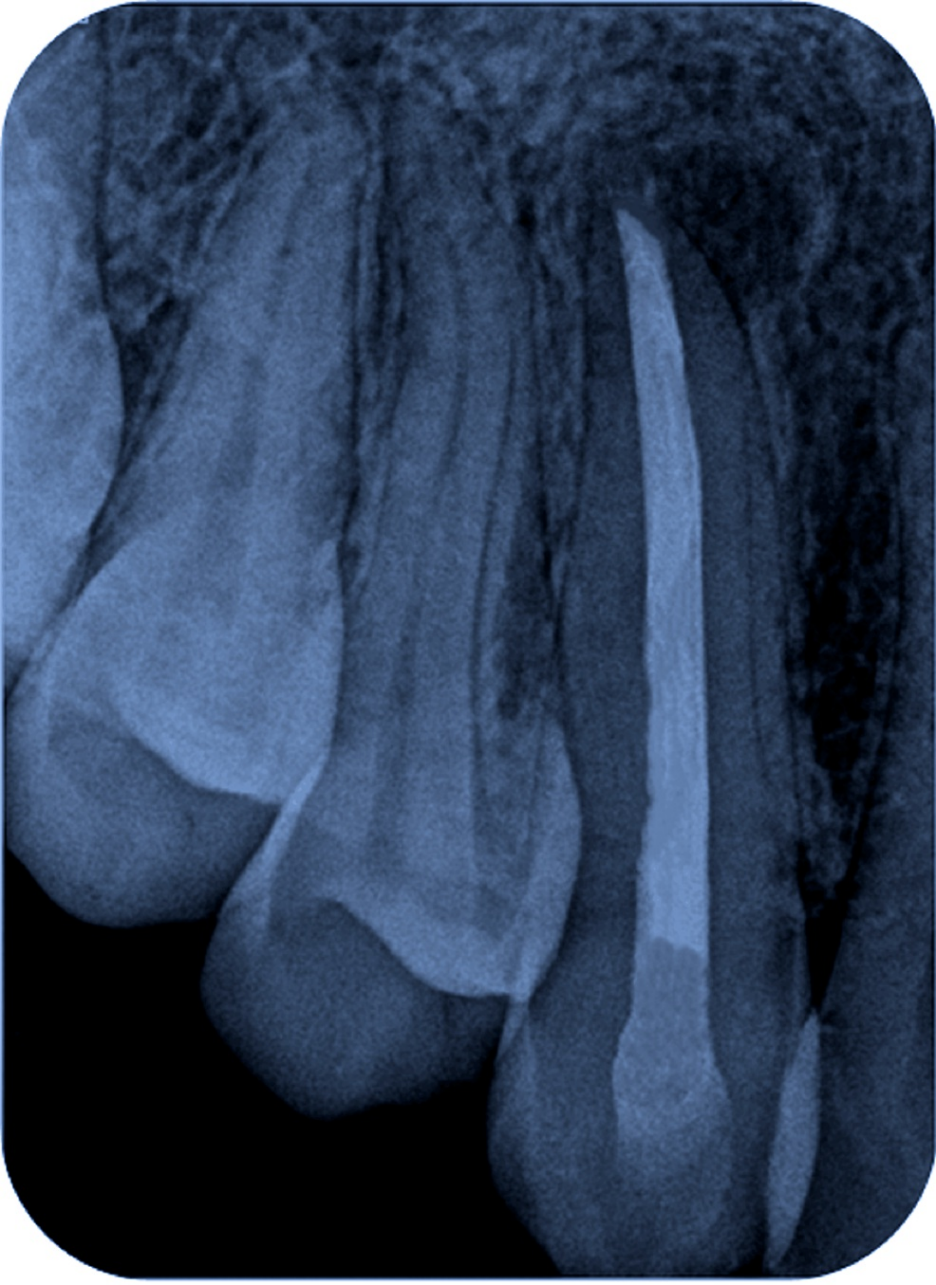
The periapical radiograph recorded at one-year follow-up. It showed a healing periapical lesion associated with tooth number 13.
